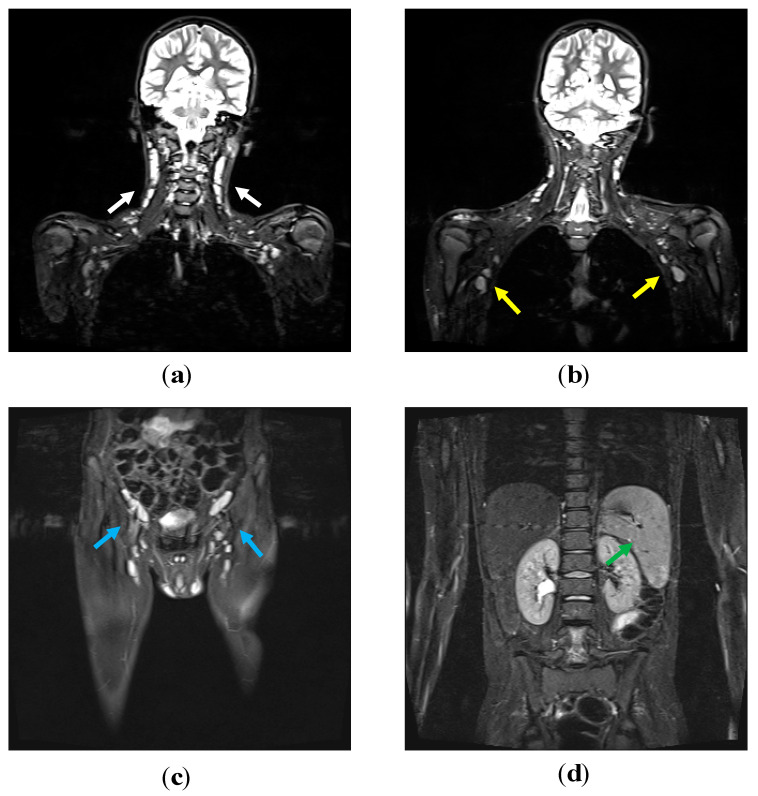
P5′s whole-body magnetic resonance imaging (MRI). On coronal fat suppressed T2 weighted sequences, numerous homogeneously hyperintense lymph nodes are detectable at the level of the neck (a) (white arrows), the axillary regions (b) (yellow arrows) and in the inguinal area bilaterally (c) (blue arrows). The spleen has a homogeneous signal intensity and increased size (d) (green arrow).
